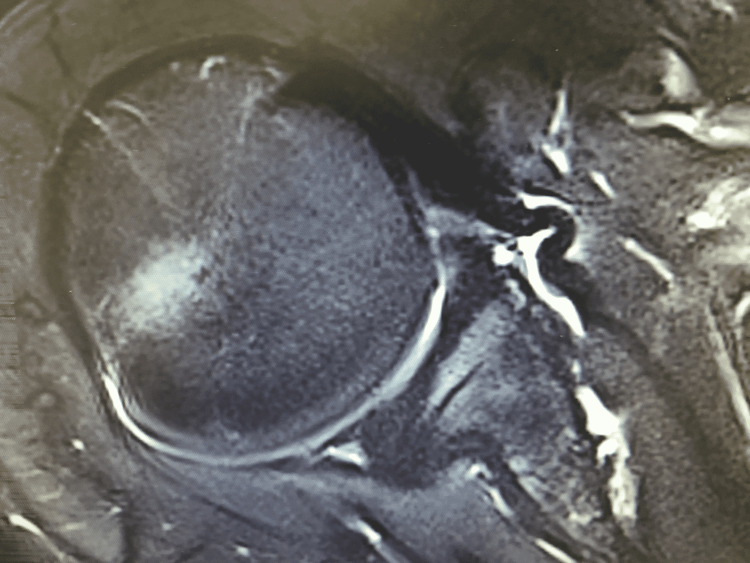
MRI scan showing a significant reverse Bankart lesion with a loose subscapularis tendon
